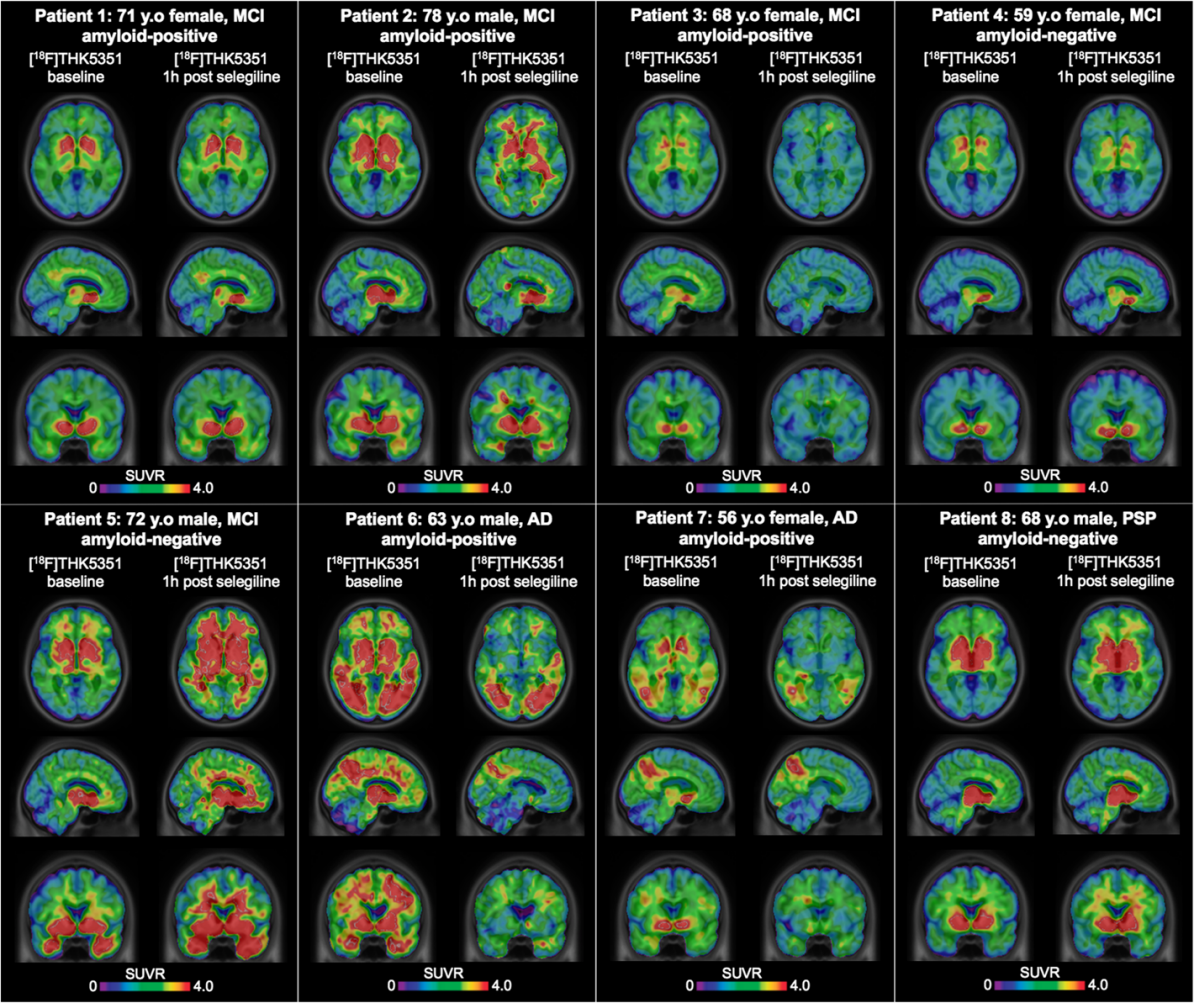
The effects of selegiline in cerebellum mask the reduction of ^18^F-THK5351 standardized uptake value ratio. Standardized uptake value ratio (*SUVR*) map overlaid on a structural MRI scan showing the baseline and 1 h post-selegiline SUVR from 50 to 70 min after ^18^F-THK5351 injection in the eight patients. *AD* Alzheimer’s disease, *MCI* mild cognitive impairment, *PSP* progressive supranuclear palsy, *y.o* years old
